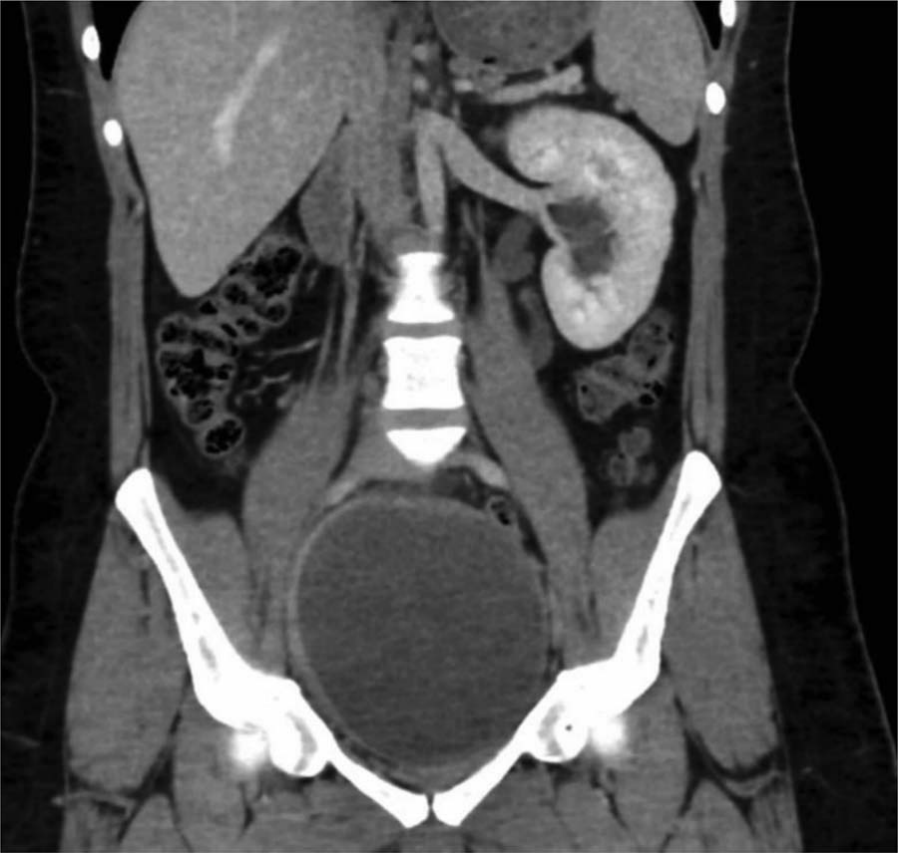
Coronal CT scan showing an enlarged right seminal vesicle cyst measuring 8.2 × 6.4 × 5.1 cm and ipsilateral renal agenesis.
